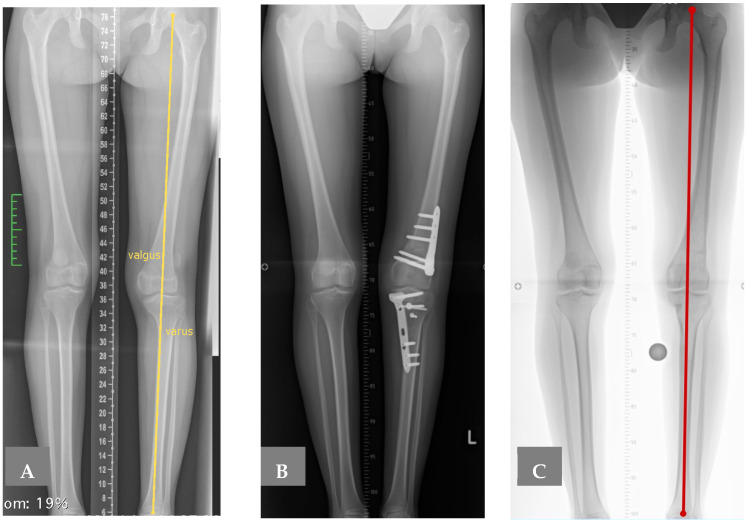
Anteroposterior radiographs showing (A) valgus deformity of the distal femur and varus deformity of the proximal tibia before surgery, (B) osteotomy plates and screws eight weeks after surgery, and (C) straight femur and tibia nine months after osteosynthetic material was removed.
